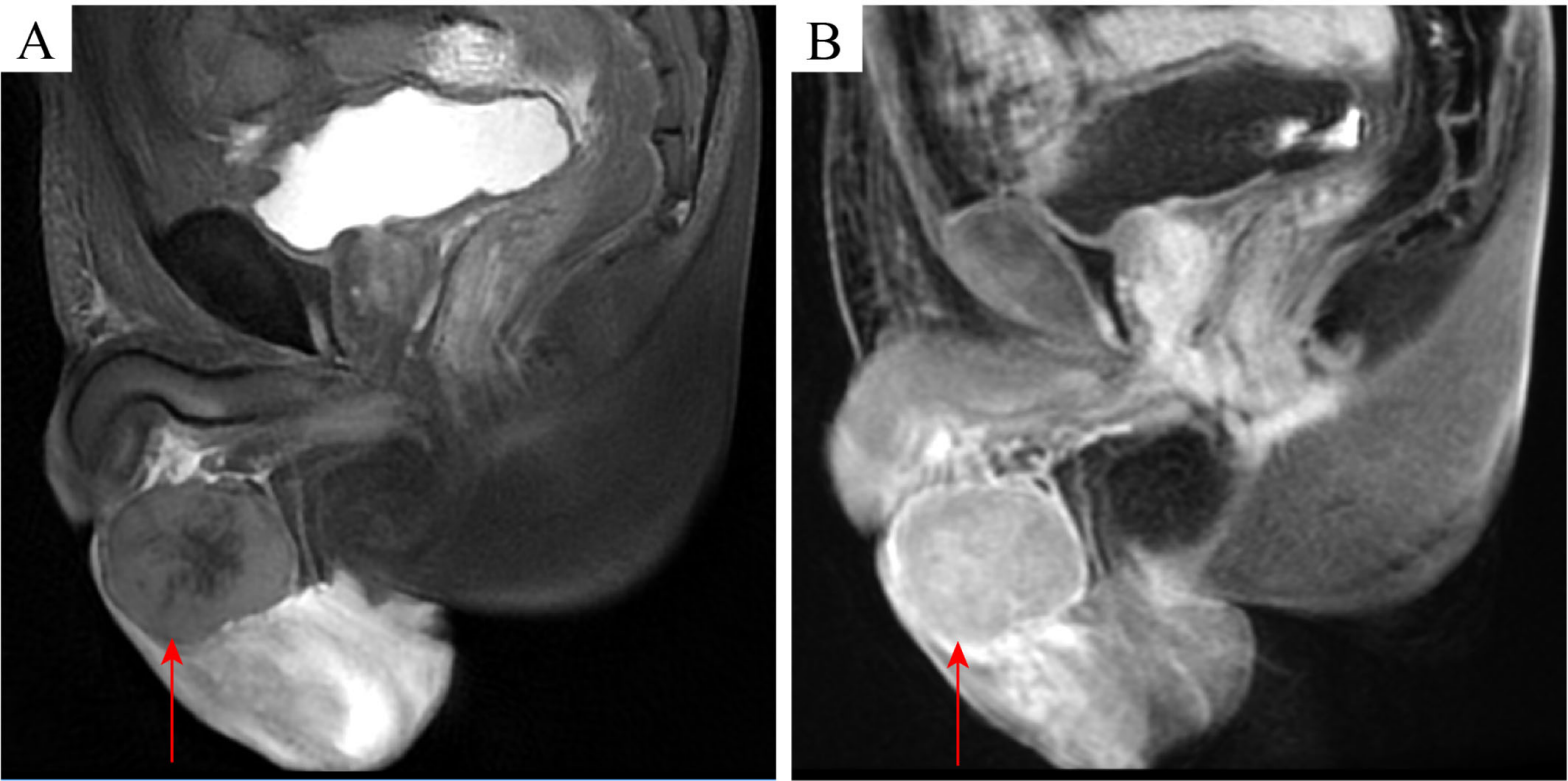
Pelvic MRI of the left scrotal mass. (A) T2-weighted image demonstrates a heterogeneous cystic-solid mass (52 mm × 56 mm × 50 mm) in the left epididymal region (arrow), with irregular margins and mixed signal intensity. (B) Contrast-enhanced T1-weighted image reveals marked peripheral rim enhancement of the mass (arrow), with unclear boundaries between the inferior margin of the mass and the left testis.
